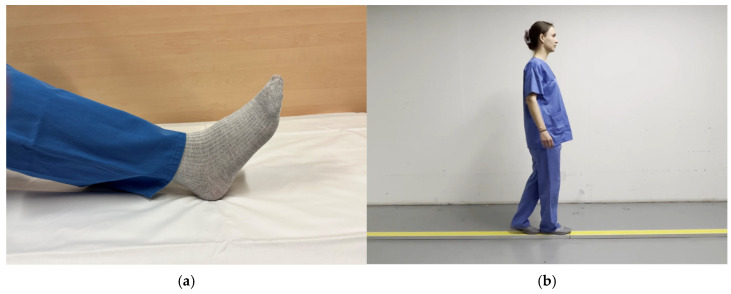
Functional magnetic resonance imaging paradigm—task activation: (a) image to prompt the foot tapping task; (b) video to prompt MI of the balancing task.
